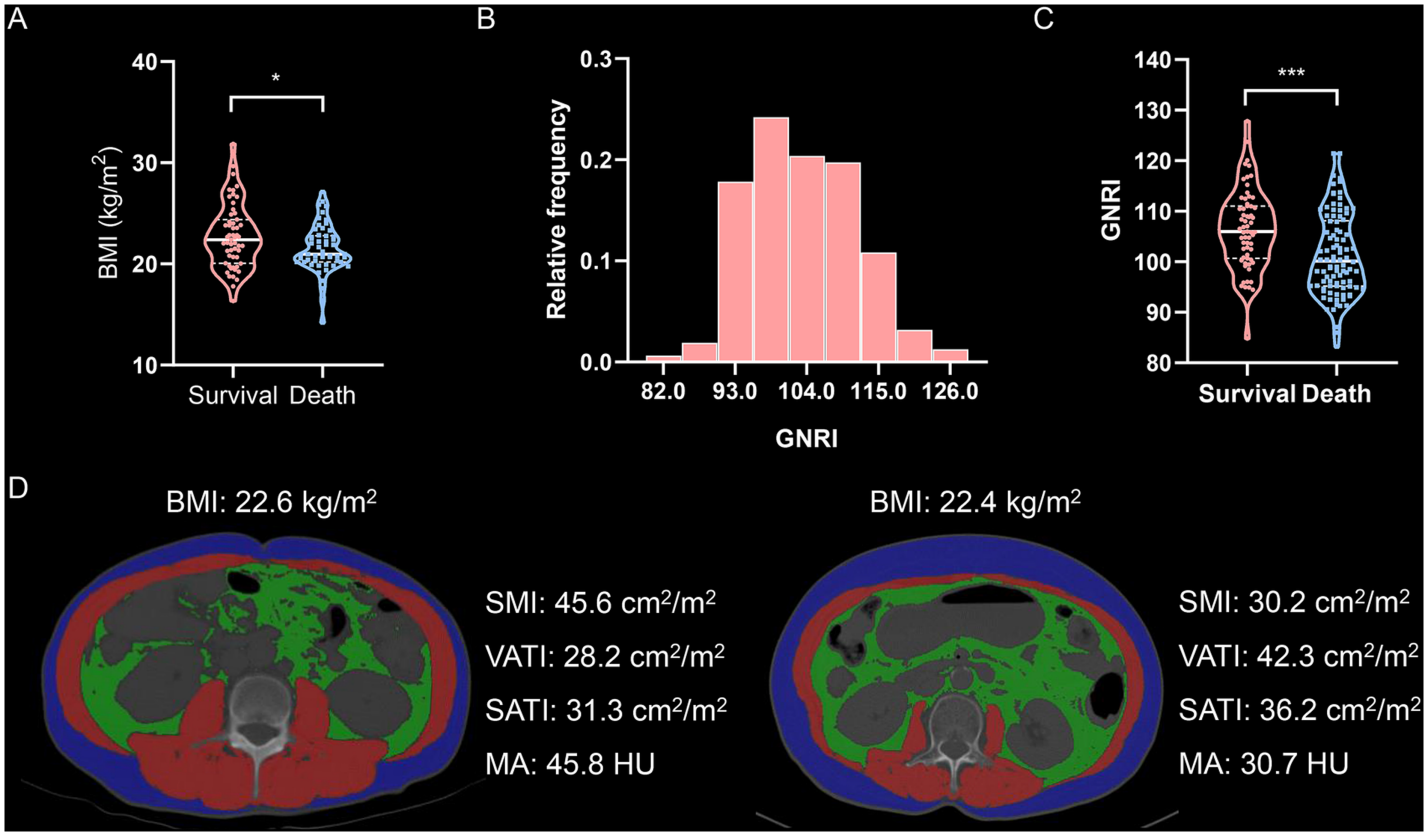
Body compositions quantified by cross-sectional computed tomography images of the third lumbar vertebra. (A) Violin plot shows BMI values in survival or death. (B) Distribution of GNRI values. (C) Violin plot shows GNRI values in survival or death. (D) Cross-sectional computed tomographic images at the level of the third lumbar vertebra among two patients with almost identical BMI (The red shadows represent the skeletal muscle areas, the green shadows represent the visceral adipose tissue areas, and the blue shadows represent the subcutaneous adipose tissue areas, respectively.). GNRI, geriatric nutritional risk index; SMI, skeletal muscle index; VATI, visceral adipose tissue index; SATI, subcutaneous adipose tissue index; MA, muscle attenuation.
